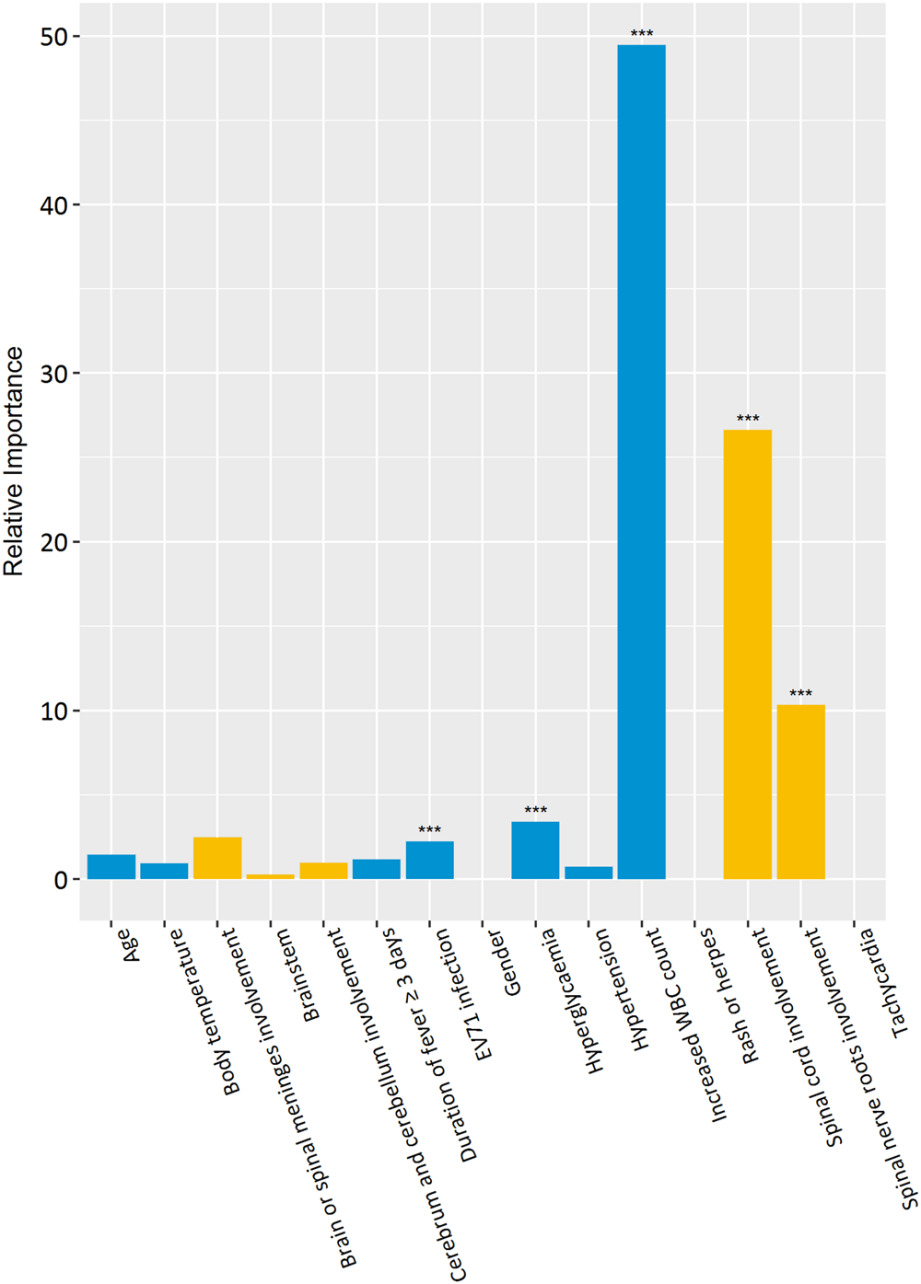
Relative importance (RI) for each individual predictor. Note that the *indicated the level of significance, p-value < 0.001. MRI-related predictors were in yellow and clinical predictors were in blue.
